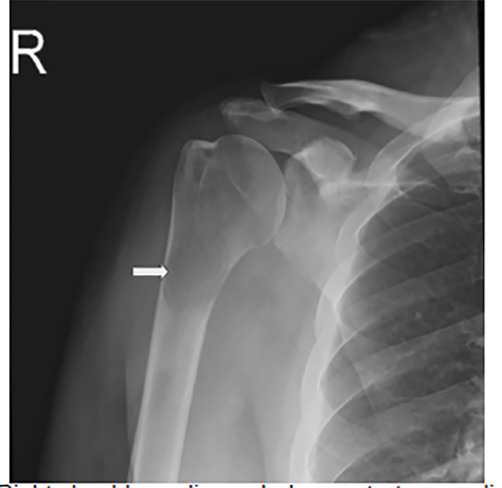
Right shoulder radiograph demonstrates a radiolucency (arrow) in the right proximal humerus on the initial visit.
